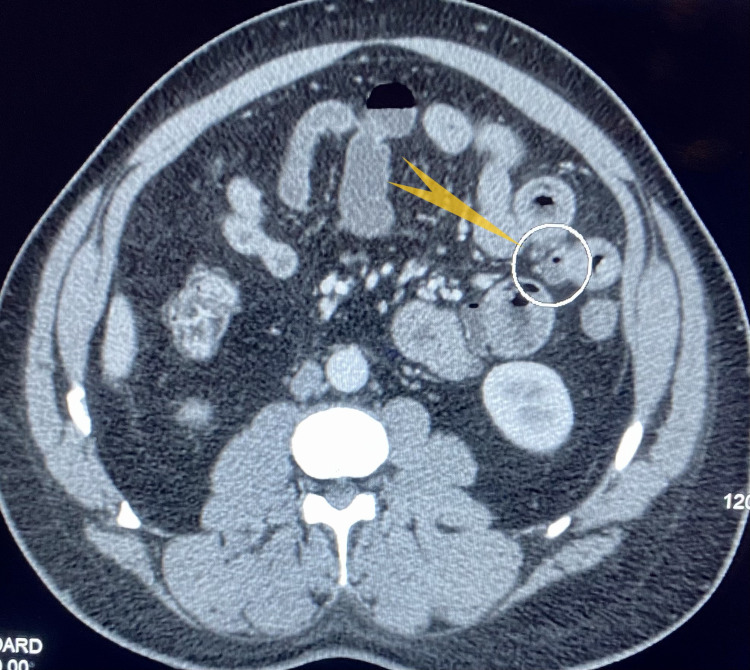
CT of the abdomen and pelvis with contrast. Showing jejunal diverticulum with thickened walls and marginal stranding of the mesenteric fat (pointed yellow arrow).
